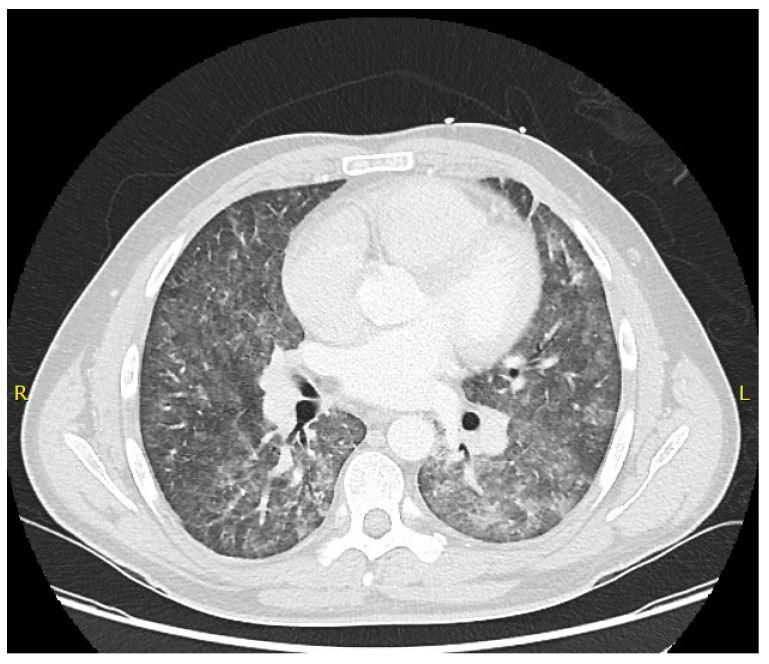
A computerized tomography (CT)-Thorax image showing centrilobular ground glass opacification in an HIV-infected patient with *Pneumocystis* pneumonia.
